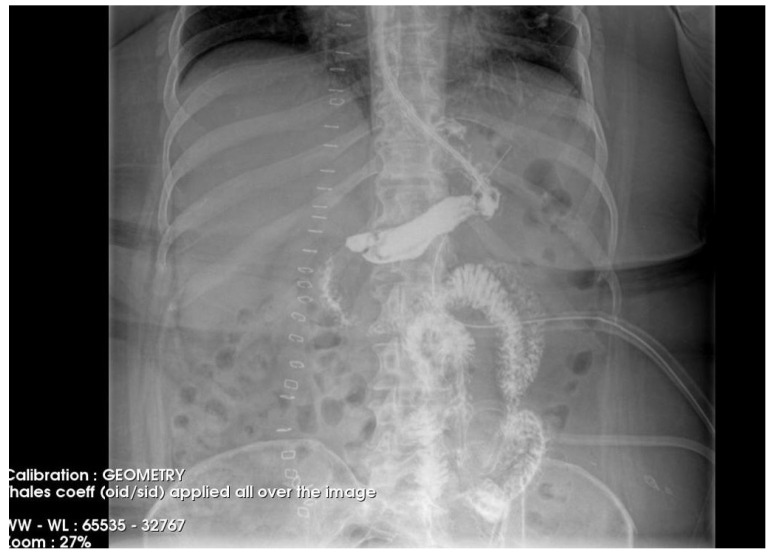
Contrast-enhanced abdominal radiograph demonstrating complete resolution of the fistulous tract, with no contrast leakage or communication visible.
